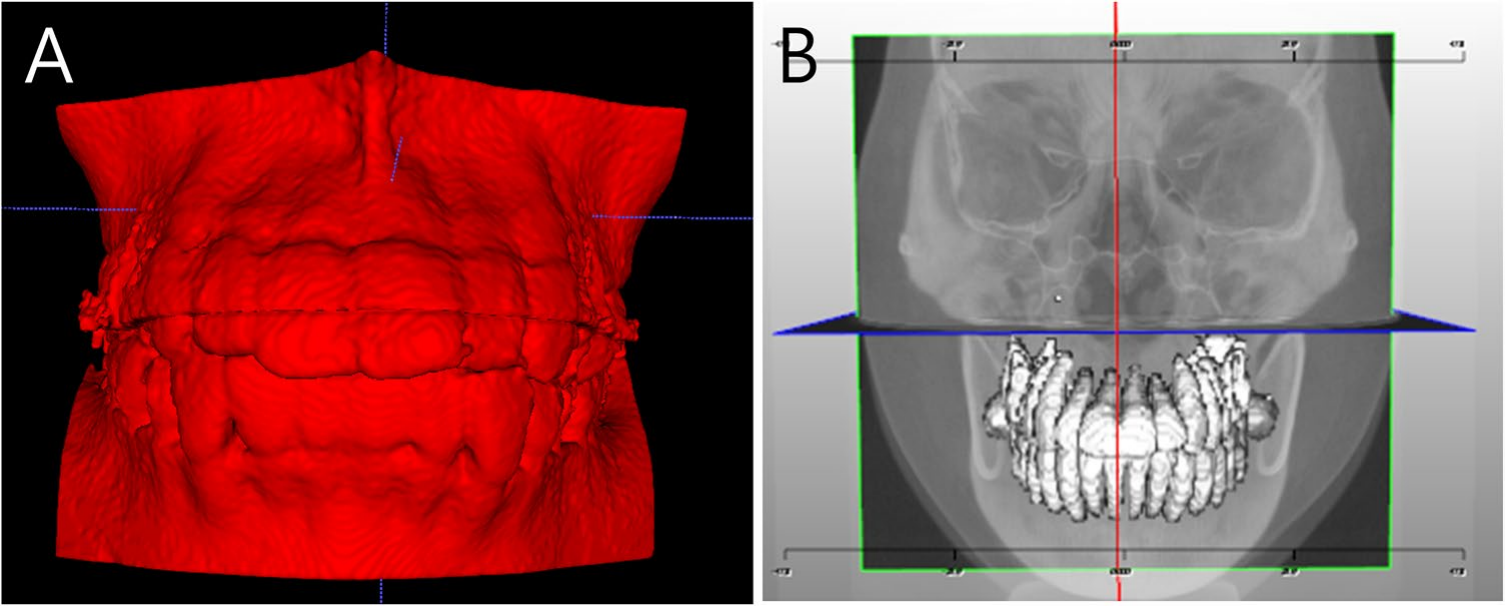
Segmentation of the maxilla, mandible, and teeth from CBCT. (A), segmentation of the maxilla and mandible. (B), segmentation of the individual teeth. CBCT, cone beam computed tomography.
